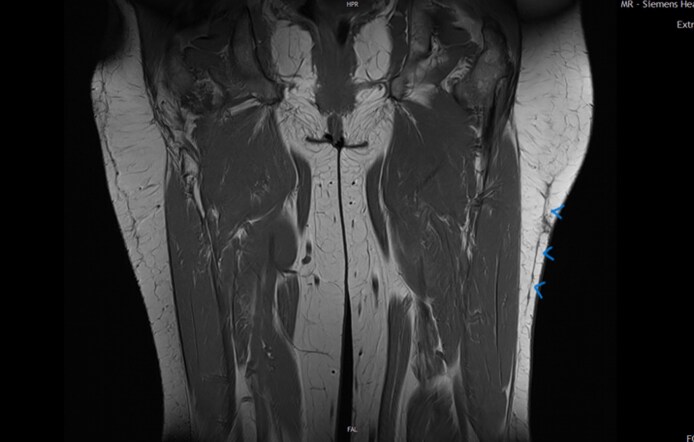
Follow-up MRI conducted 1 year after water jet–assisted liposuction of the left thigh. Arrows mark the subcutaneous cleft that remains visible in the MRI image. The reduction in subcutaneous fatty tissue volume, a linear hypointense signal suggestive of scar formation and no evidence of cavity formation.
